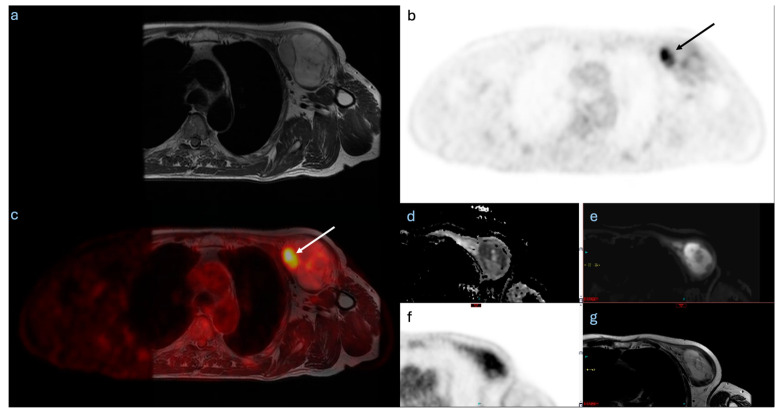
(a) Standard T1 MRI of grade 3 myxofibrosarcoma of the left chest wall, (b) FDG PET, and (c) fused PET-MRI images showing variable FDG uptake across the tumour. (d,e) Diffusion-weighted images (DWIs) and ADC. (f) FDG PET and (g) standard T1 MRI assessment of a myxofibrosarcoma tail. The black and white arrows for the FDG PET (b) and fused PET-MRI (c), respectively, identify the focus of higher FDG activity within the tumour, compatible with tumour heterogeneity and varied FDG uptake.
